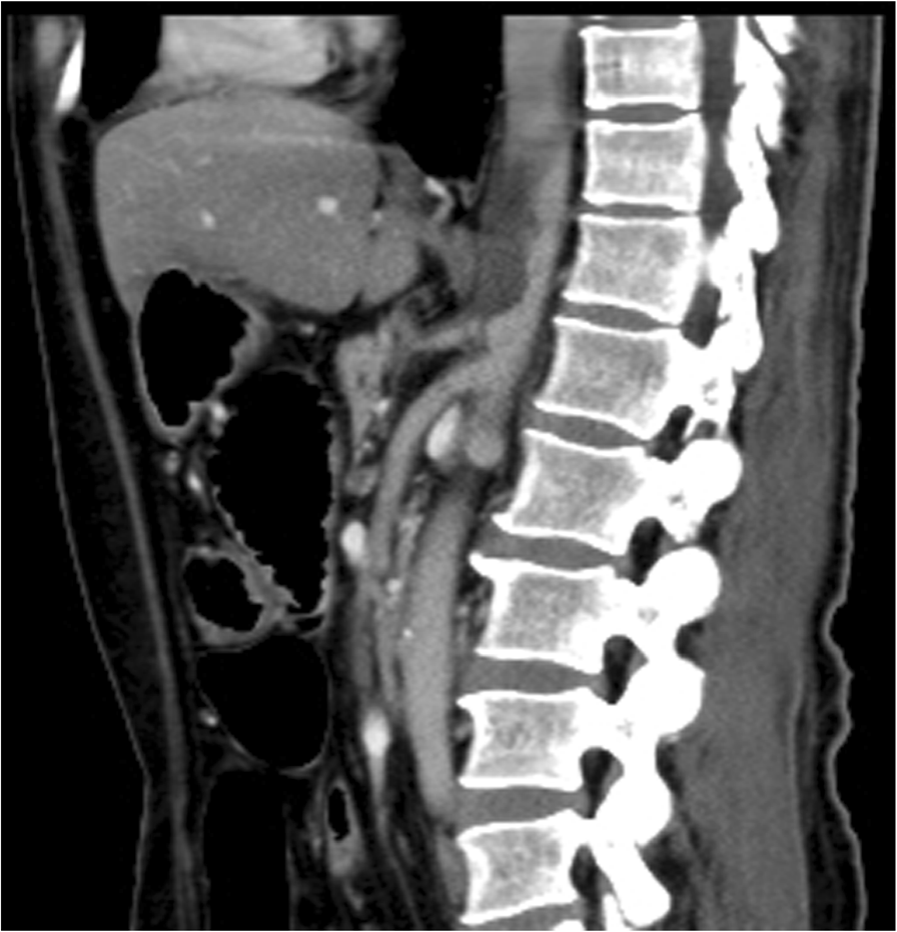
Computed tomography examination. Sagittal computed tomography scan showing aortic thrombosis
